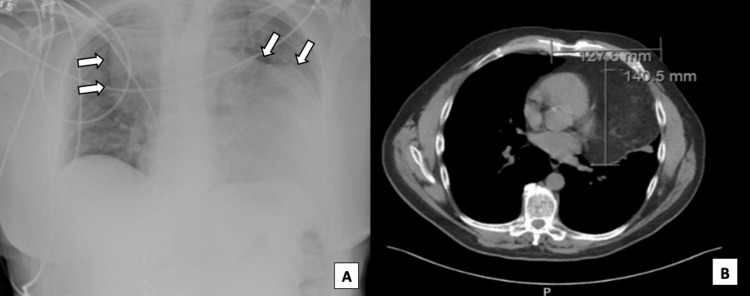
Chest Mass Imaging (A) Anteroposterior X-ray identifying large mass in the chest (white arrows) and (B) axial CT of the chest showing the 127.6 x 140.5 mm mediastinal mass abutting and compressing the left ventricle.
